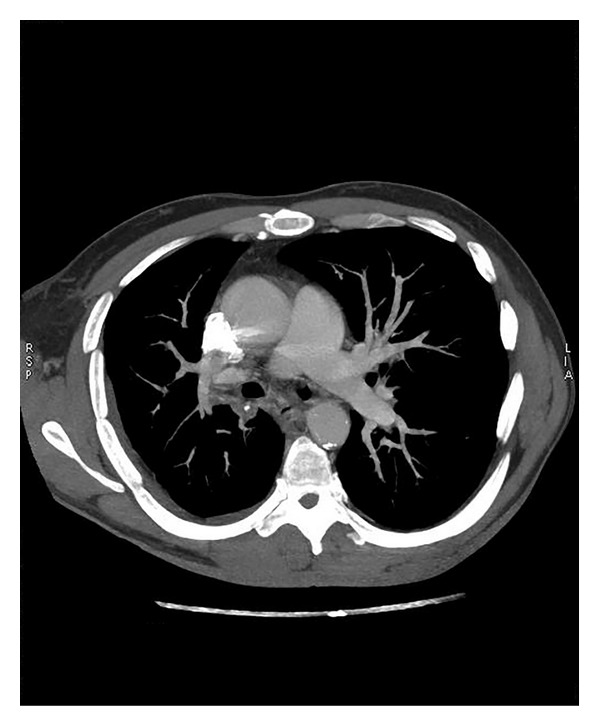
Multidetector computed tomography showed a PAPVC from the right upper lobe to the superior vena cava (arrow).
